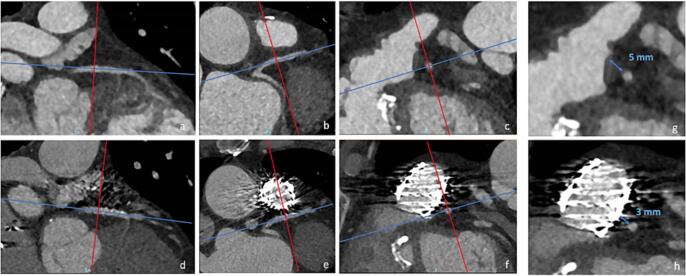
CTA before (a-c) and after PPVI (d-f). All measurements were performed using double oblique reconstructed images. Coronary artery morphology, patency and the relationship with the RVOT was assessed (g,h).
